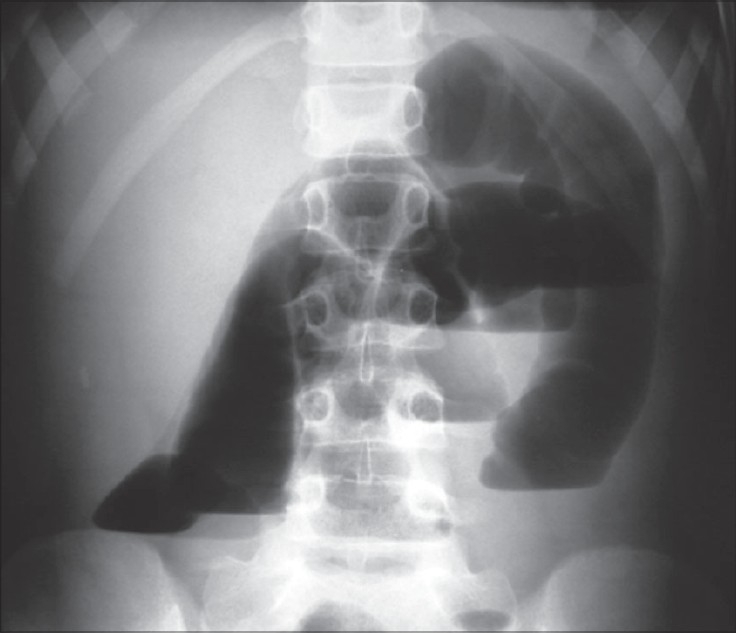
X-ray showing intestinal loops with air-fluid levels
